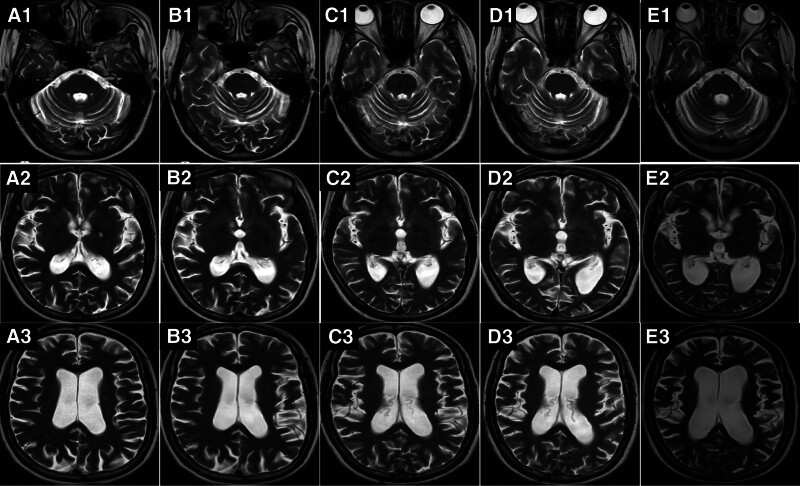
T2-weighted imaging follow-up results of the patient from 2017 to 2023. magnetic resonance imaging (MRI) data for 2017, 2018, 2019, 2020, and 2021 are coded as A1–A3, B1–B3, C1–C3, D1–D3, and E1–E3, respectively.
